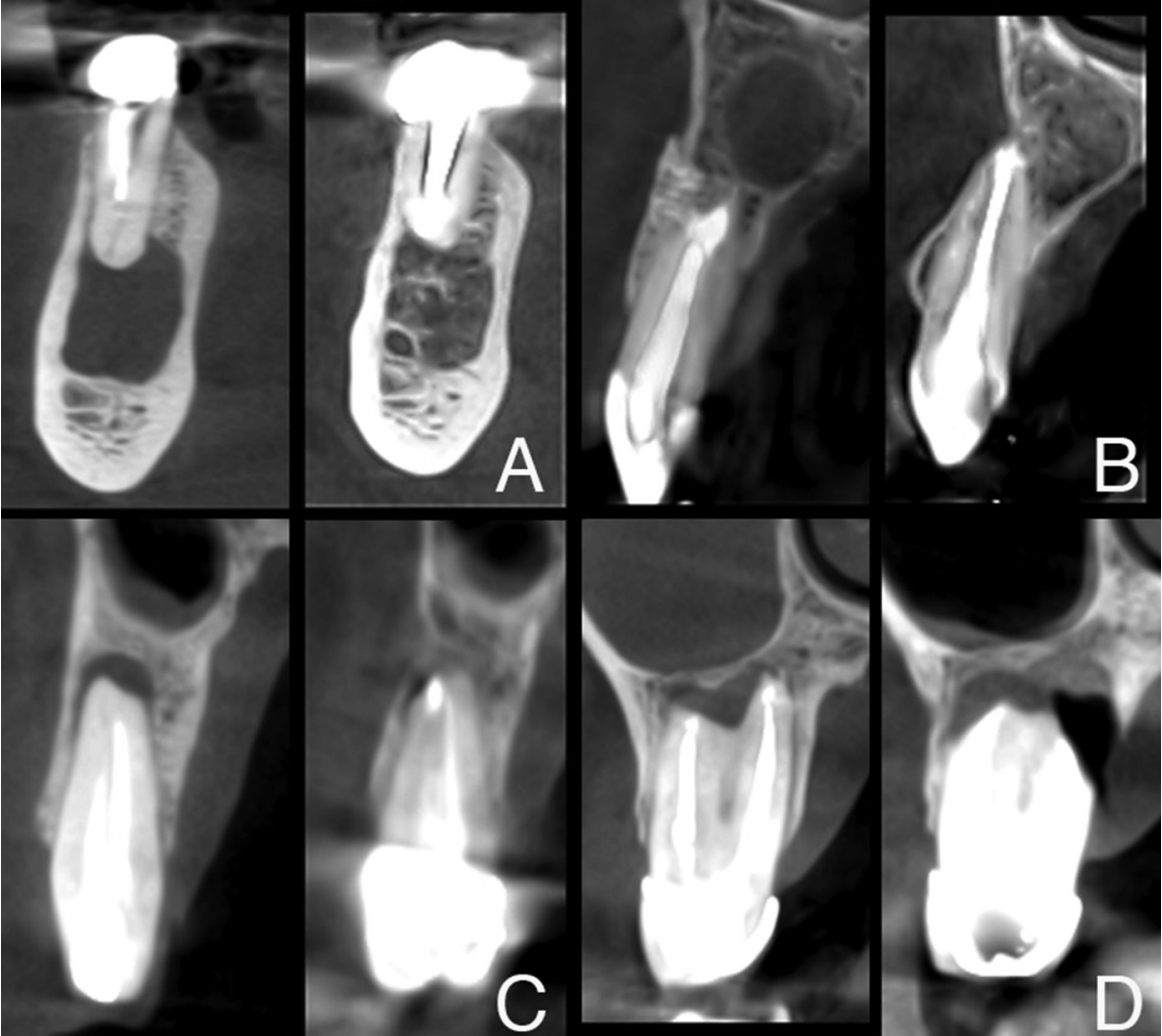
Healing of periapical lesions after endodontic microsurgery according to the modified Penn 3D criteria. A) Complete healing. B) Limited healing. C) Uncertain healing. D) Unsatisfactory healing.
